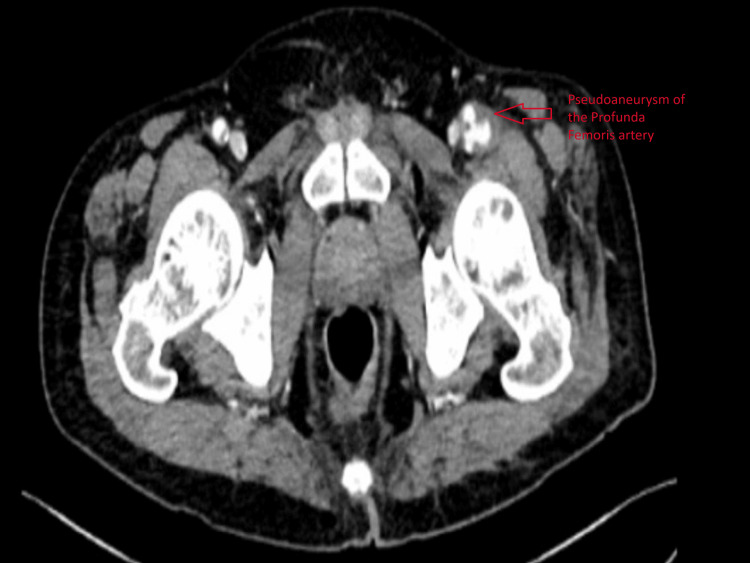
CT scan showing pseudoaneurysm of the profunda femoris artery
